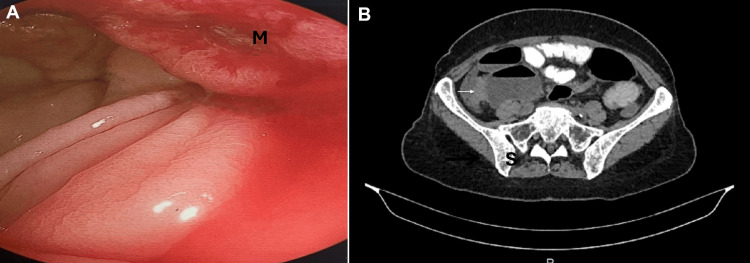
Endoscopic and imaging findings (A) Endoscopy shows a slowly oozing, ulcerated lesion representing intestinal wall infiltration by metastatic breast cancer. M: intestinal breast cancer metastasis; I: normal intestinal mucosa. (B) CT scan of abdomen and pelvis with oral and IV contrast showing a space-occupying lesion obstructing the ileocecal valve. Black arrow: ileocecal valve obstruction. S: dilated proximal small intestine loops
